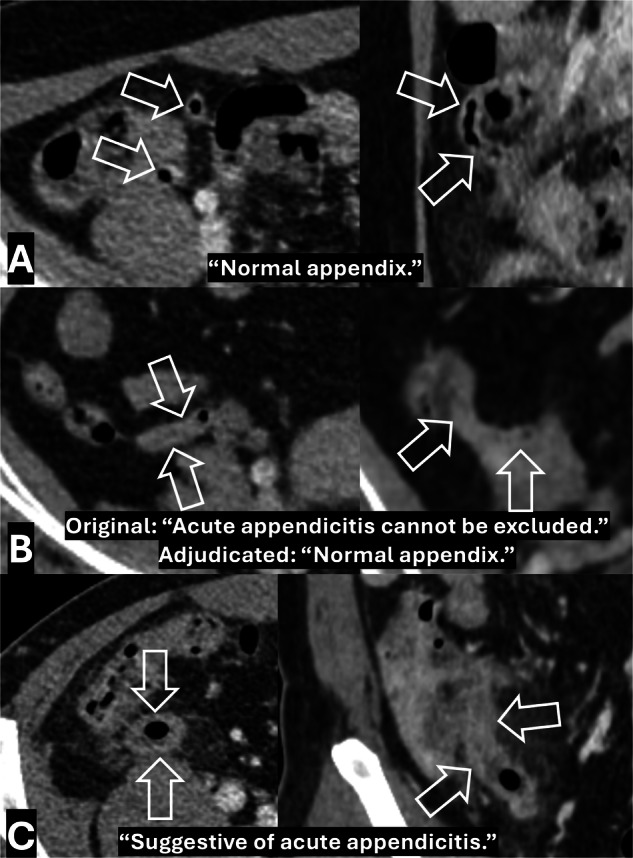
Examples of cases with (A) a normal appendix and unambiguous report language “normal appendix,” (B) borderline-sized appendix (7 mm; lack of air filling) and ambiguous report language “acute appendicitis cannot be excluded” that had been adjudicated as “normal appendix,” and (C) acute appendicitis and unambiguous report language “suggestive of acute appendicitis.” It should be emphasized that the adjudicators reviewed only the CT report for the purpose of adjudication but not these images. Arrows = appendices
